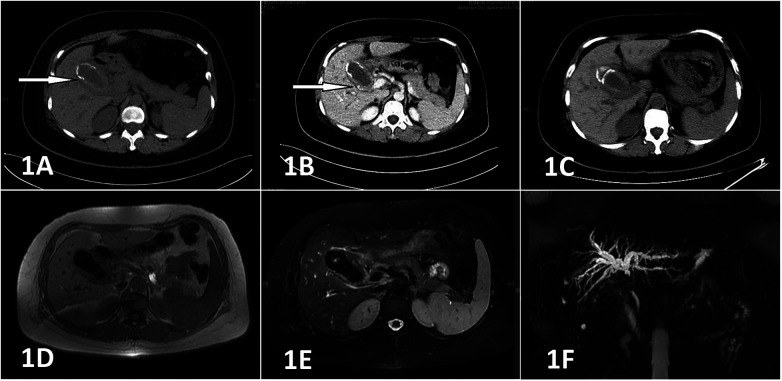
Initial plain CT scan shows an enlarged gallbladder with thickened walls and multiple ring-like hyperdense lesions within [(A), ↑]. Contrast-enhanced CT demonstrates heterogeneous wall enhancement [(B), ↑]. Follow-up plain CT scan shows worsened blurring of the surrounding fatty margins (C). Plain MRI scan with T1-weighted imaging reveals a thickened gallbladder wall with low signal intensity within (D); T2-weighted imaging demonstrates a gallbladder filled with short T2 signal intensity and the thickened neck wall contains cord-like low signal intensity (E). MRCP shows intrahepatic biliary dilatation with abrupt narrowing of the common bile duct, suggestive of Mirizzi syndrome (F).
